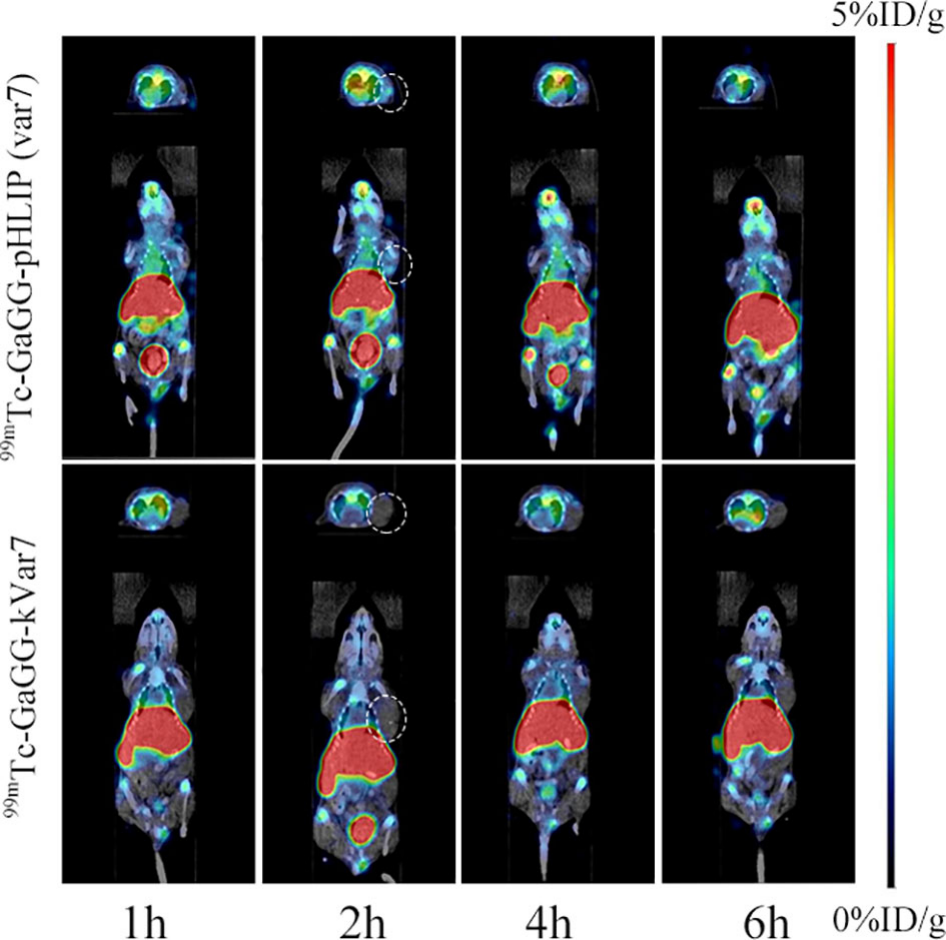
SPECT/CT imaging of ^99m^Tc-pHLIP (Var7) and ^99m^Tc-kVar7 in the MDA-MB-231 tumor model (n=3).
